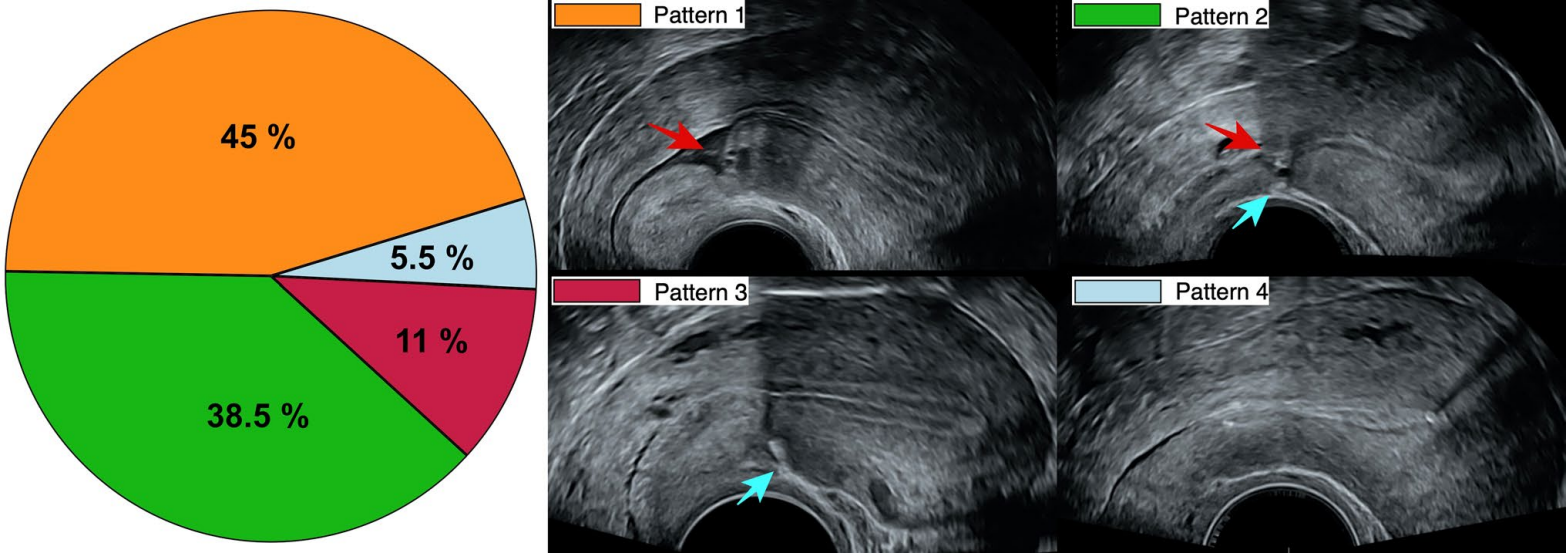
Pie chart representation of the CS scar patterns; pattern (1) with isolated niches, pattern (2) with simultaneous niches and fibrosis, pattern (3) with isolated fibrosis, and pattern (4) lacking myometrial defects. The sonographic appearance of niches and fibrosis is shown with red and blue arrows, respectively
